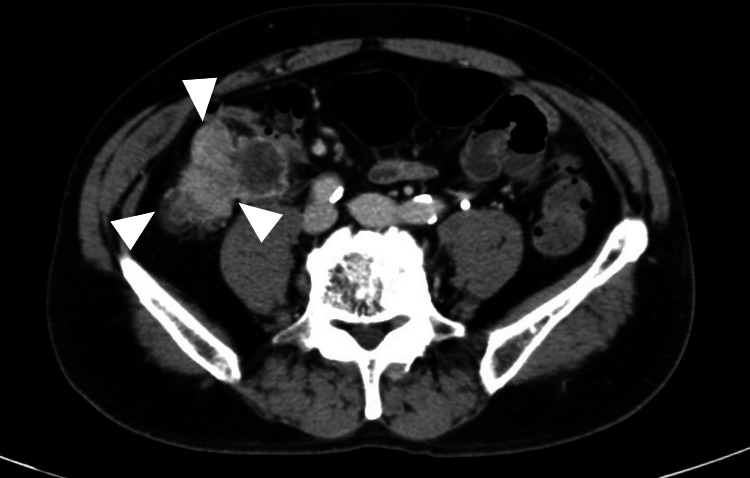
Contrast-enhanced CT of the abdomen Tumor formation with contrast effect was observed in the ileocecal region.
